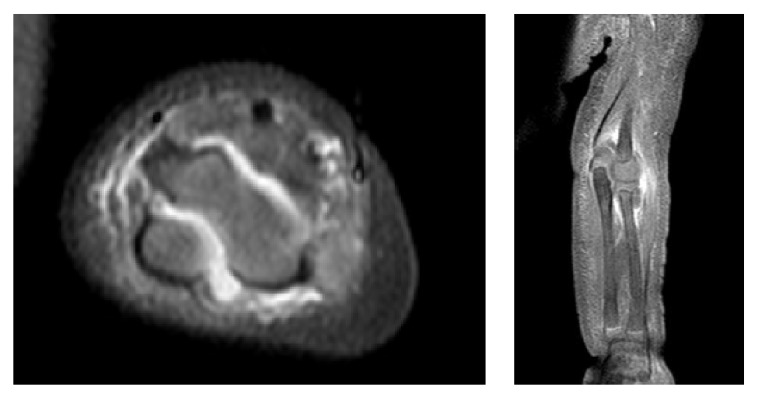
MRI of the left elbow reveals a large effusion between the humerus and olecranon process of the ulna on axial view and cellulitis and myositis on coronal view. Osteomyelitis was not detected.
